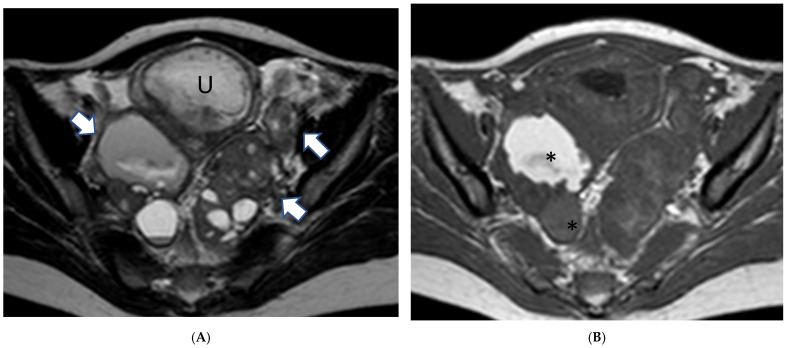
A 29-year-old woman at gestational week 9 presented with abdominal distention and fatigue: (A) Axial T2-weighted image demonstrates bilateral ovarian masses (arrows) with cystic and solid components. (B) Corresponding axial T1-weighted image shows high signal intensity content within some of the cystic compartments (*) indicative of hemorrhagic or high protein fluid. Low-grade serous cystadenocarcinoma of both ovaries was confirmed during surgery. U: gravid uterus.
